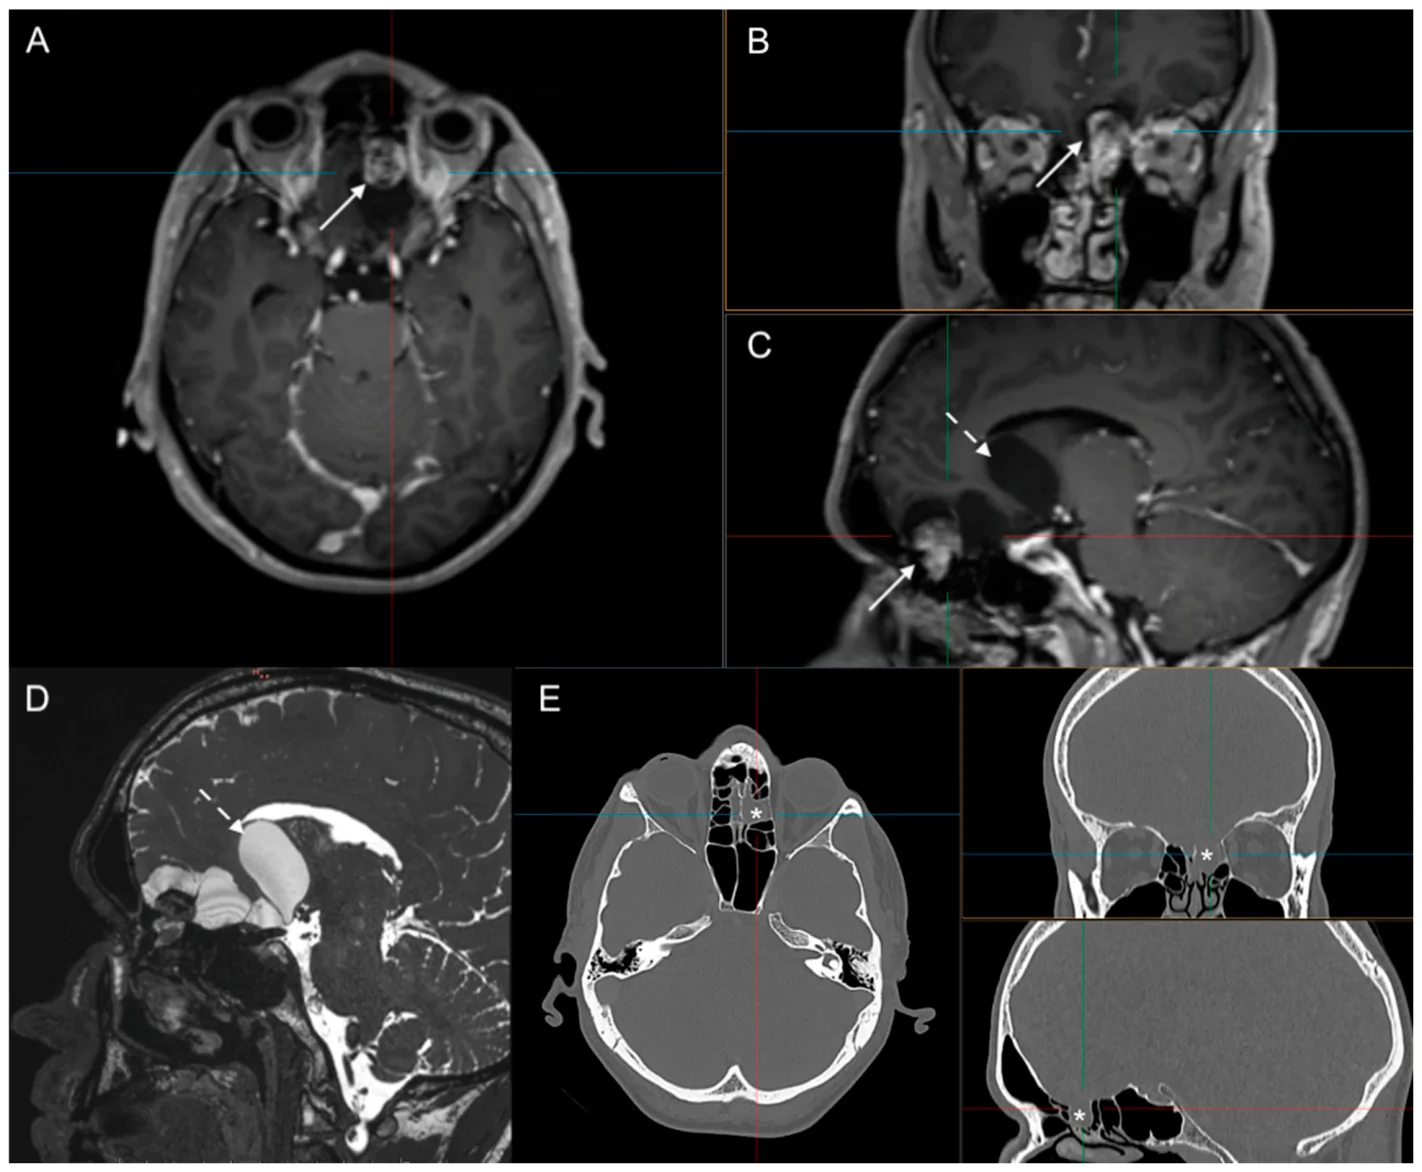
Preoperative imaging of the olfactory groove schwannoma, showing the solid component of the lesion (arrow), the cystic component (dashed arrow) and ethmoidal bone erosion (asterisk). (A) Axial T1-weighted post-contrast image MRI. (B) Coronal T1-weighted post-contrast MRI. (C) Sagittal T1-weighted post-contrast MRI. (D) Sagittal CISS sequence MRI. (E) Bone window CT scan.
